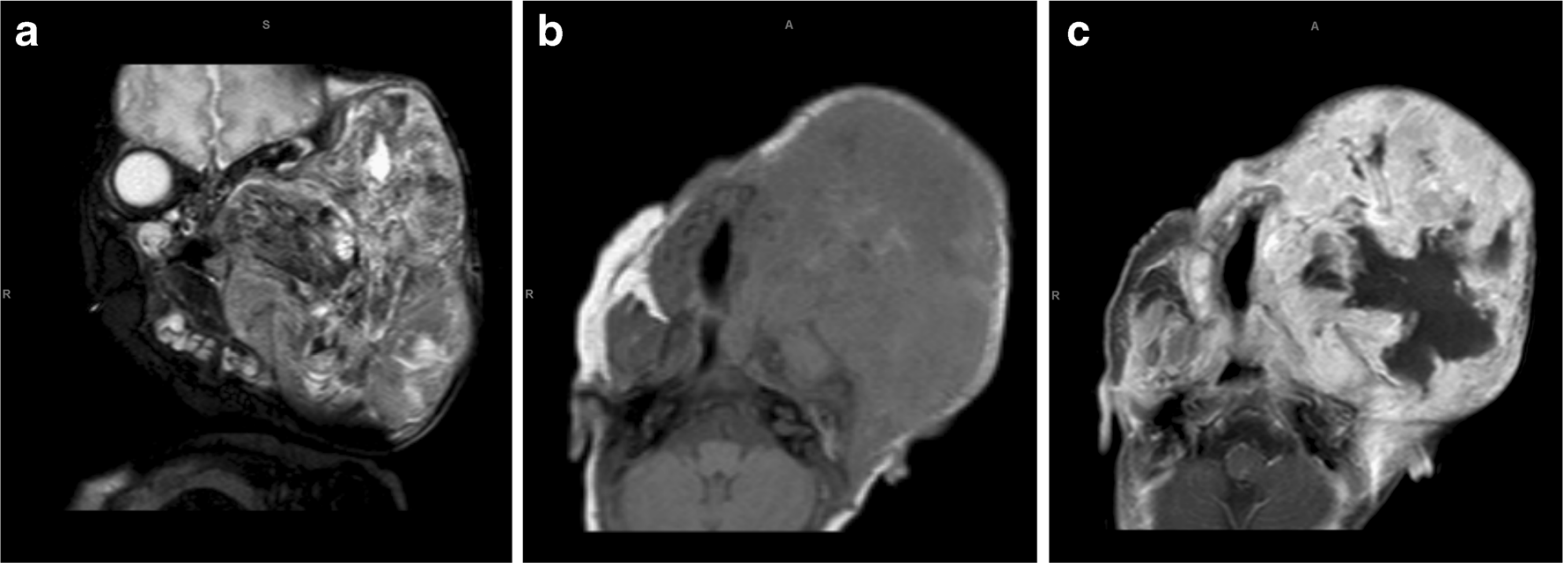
Congenital rhabdomyosarcoma. Coronal T2-W (a) and axial T1-W (b) MRI images show a 10 × 10.5 cm heterogeneous mass centred within the left parapharyngeal soft tissues. The tumour extends from the middle cranial fossa to the level of C4 inferiorly, with associated destruction of the left ramus of the mandible and the left lateral orbital wall. On the post-contrast T1-W image (c), there is avid enhancement with a large area of necrosis centrally
